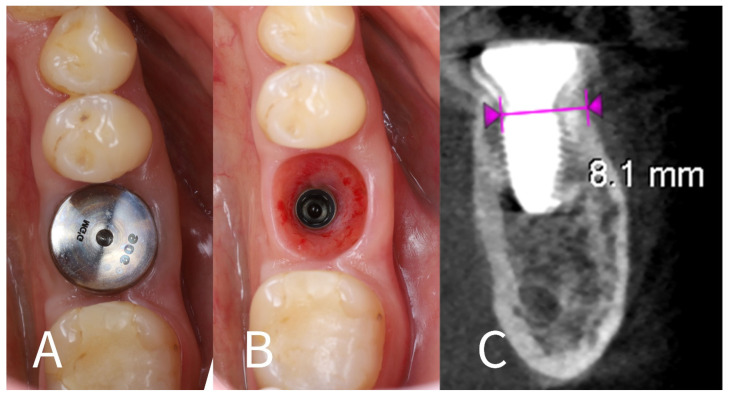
Four-month follow-up. (A)—Intraoral view at 4 months post-op, showing that the buccal gingival margin (arrow) remains at the same level as immediately after surgery, while the lingual side shows slight recession; (B)—Healthy and well-adapted soft tissues after removal of the healing cap at 4 months; (C)—4-month post-op CBCT cross-section, indicating minimal horizontal bone resorption (~0.2 mm) on the buccal aspect (measurements between violet arrowheads) due to the socket shield.
